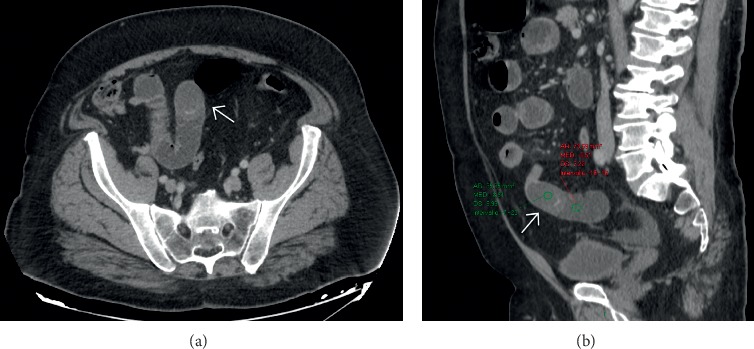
Contrast-enhanced computed tomography in a surgically proven case of gallstone ileus, in which the presence of an isodense gallstone (white arrows in (a) and (b)) in the jejunum was overlooked. Sagittal reformatted image (b) demonstrates a focal widening of the jejunum just before the transition point; this mass effect is associated with the presence of different attenuation values compared with the fluid-filled proximal bowel.
